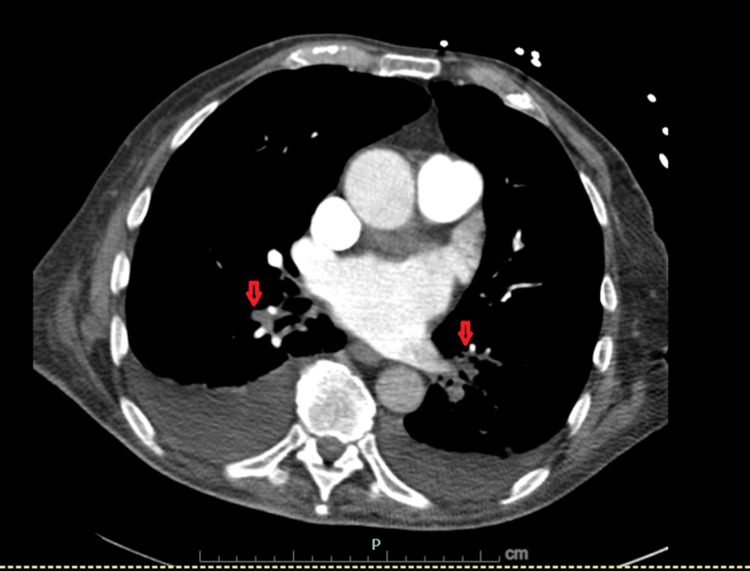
CTPA demonstrating bilateral segmental pulmonary emboli CTPA showing filling defects (red arrows) within the left lower lobe medial basal and right lower lobe lateral basal segmental branches, consistent with bilateral pulmonary emboli. Pulmonary artery caliber is normal. CTPA, CT pulmonary angiogram
